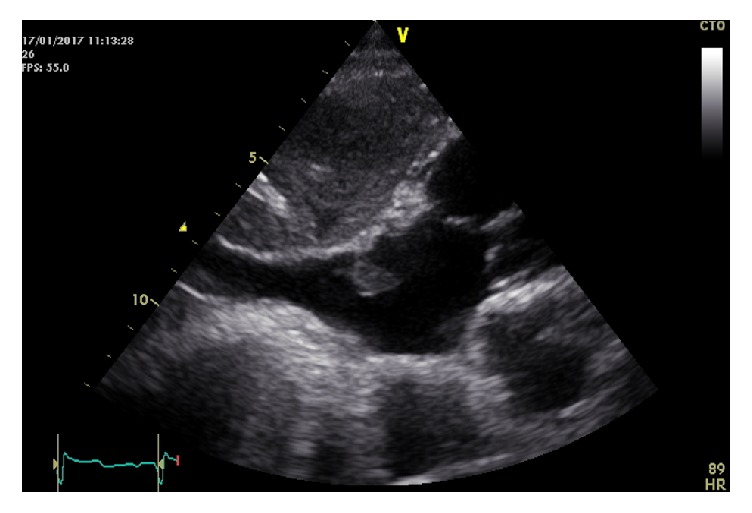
Transthoracic echocardiogram (subcostal window) with mobile vegetation attached to Eustachian valve.
